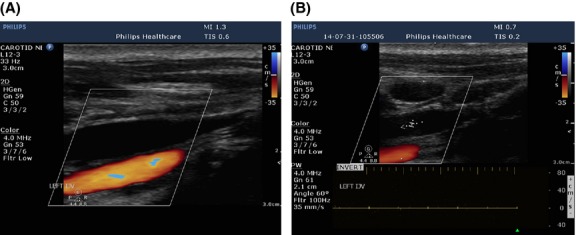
Flow not-Doppler detectable in the Internal Jugular Vein (Criterion IV) in horizontal color-flow image before (A) and after (B) spectral interrogation.
